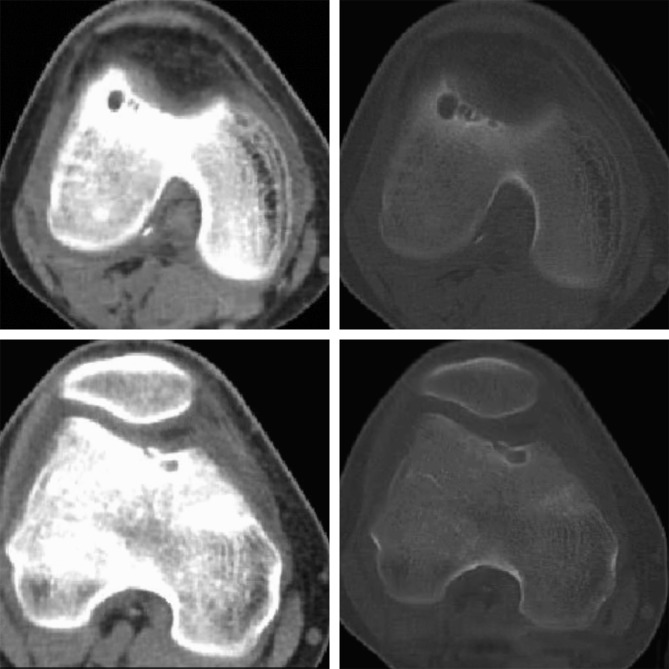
Knee CT scan image. Cystic lesions both in the lateral and medial sides of the femoral trochlea.
